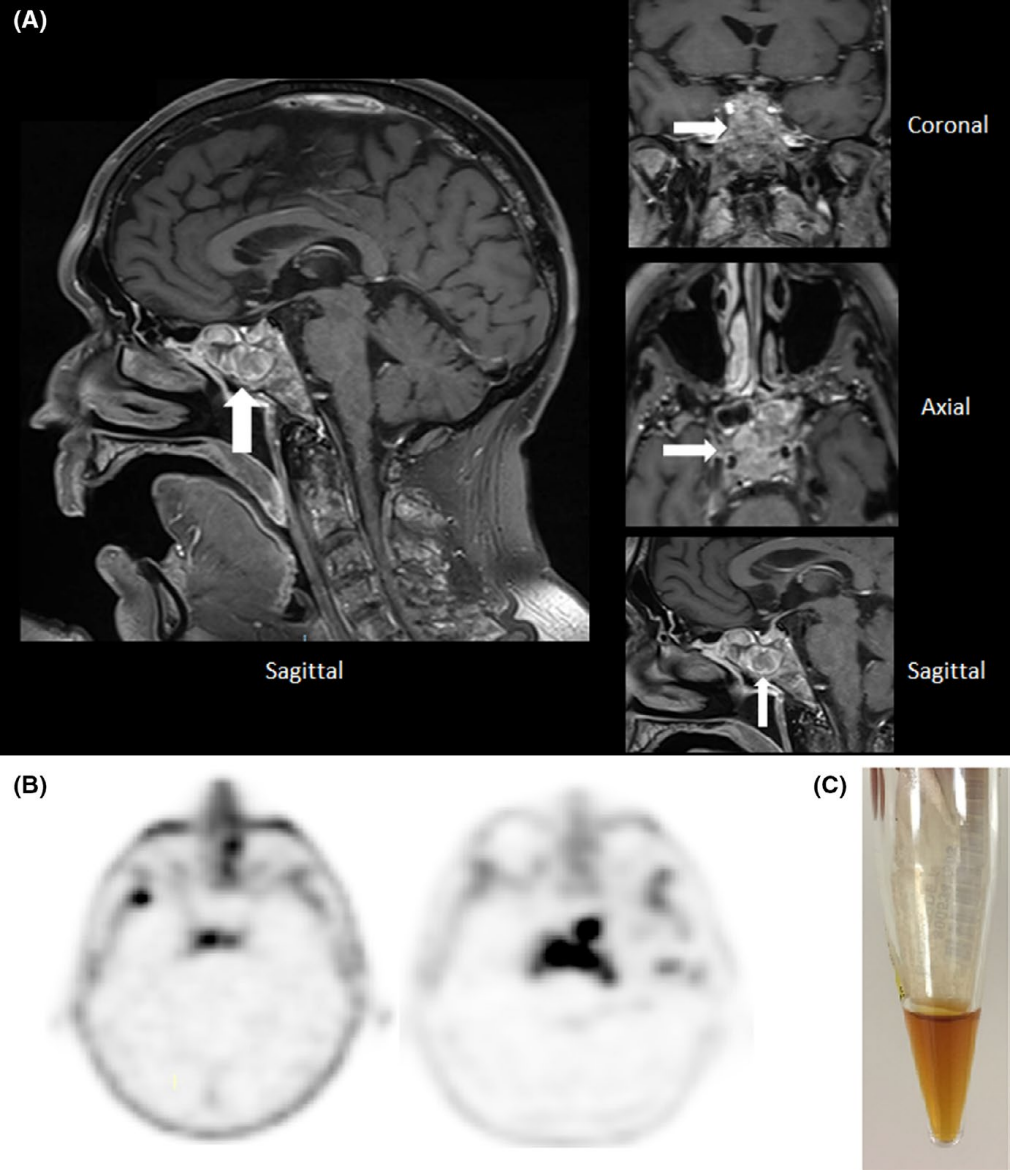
A, Cerebral MRI: sagittal T1 global sequence on the left and three dimensional T1 sequences (clivus zoom) on the right, showing a hypersignal due to an extensive process of the clivus spreading to the cavernous and sphenoidal sinuses and internal carotid sheathing. B, Choline‐PET‐CT scans showing hyperfixation of the clivus increasing between June 2017 (left) and October 2017 (right). C, Macroscopic appearance of the cerebrospinal fluid: a xantochromic honey‐like liquid similar to the serum
